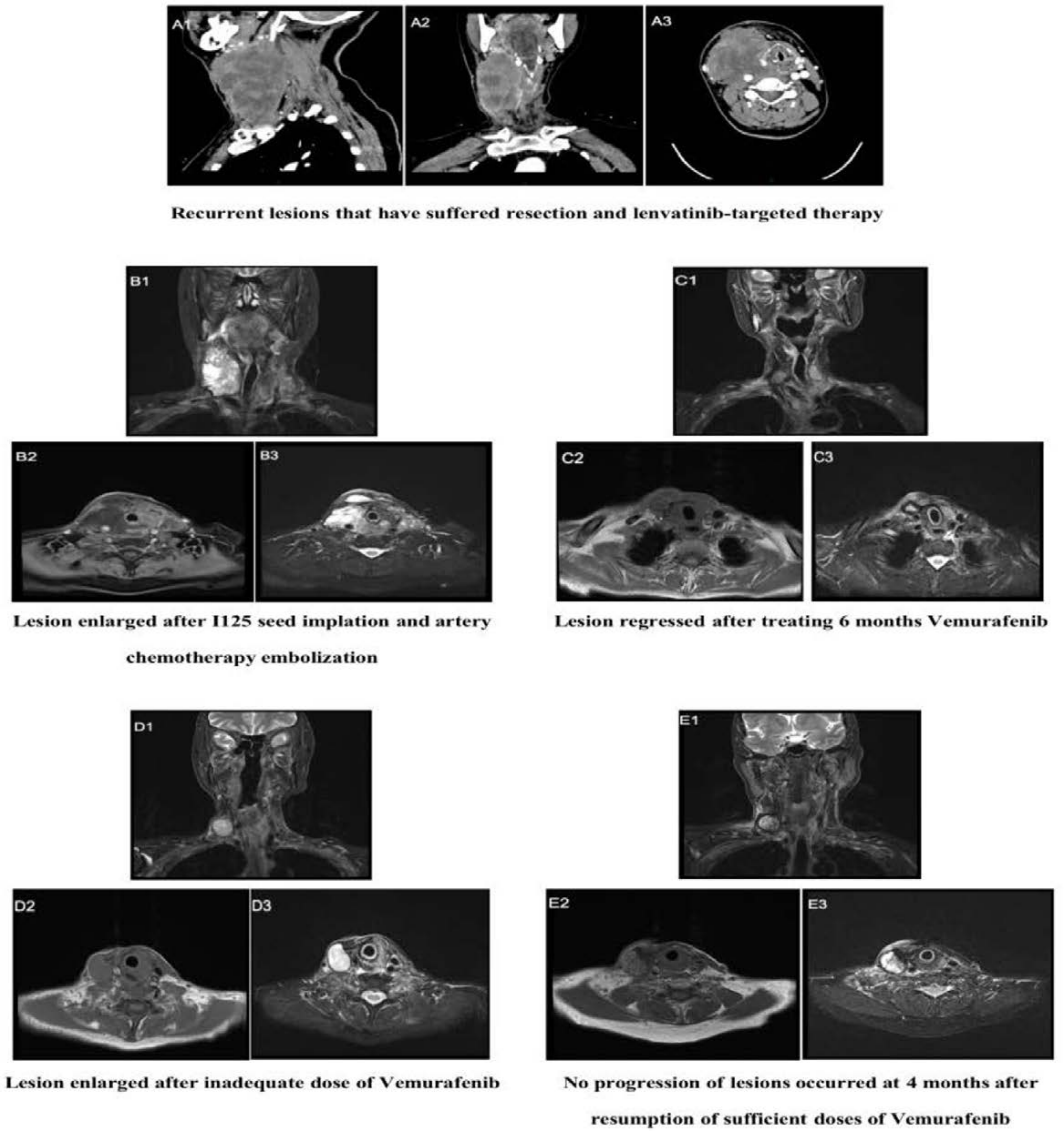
(A) Baseline CT scan of the thyroid conducted in February 2022; lesion dimensions were 45 × 58 × 101 mm. (B) Subsequent MRI post-I125 seed implantation and arterial chemoembolization in April 2022 demonstrated a lesion measuring 42 × 35 × 72 mm. (C) MRI evaluation after 6 months of vemurafenib therapy in November 2023 revealed significant tumor reduction to dimensions of 8 × 4 × 2 mm. (D) MRI obtained in February 2023 post-limited vemurafenib administration indicated lesion dimensions of 29 × 21 × 25 mm. (E) MRI conducted in June 2023, following the reinitiation of optimal vemurafenib dosing, indicated further tumor response.
